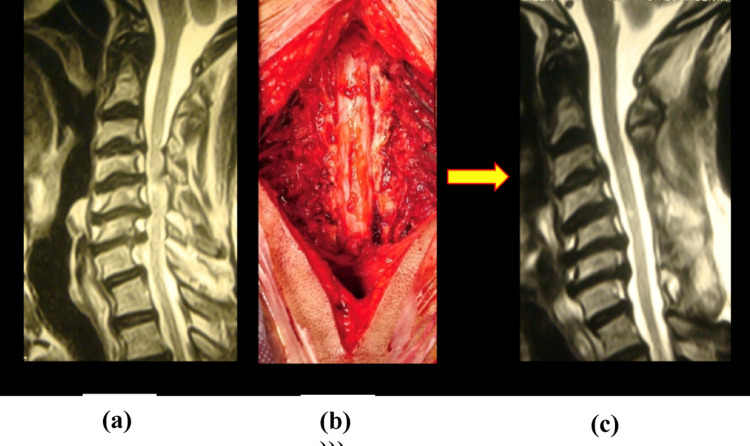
Preoperative sagittal T2-weighted cervical MRI demonstrating severe spinal cord compression with intramedullary signal change (a). Intraoperative photograph showing posterior cervical decompression (b). Postoperative sagittal T2-weighted MRI demonstrating adequate spinal cord decompression following surgery (c)
